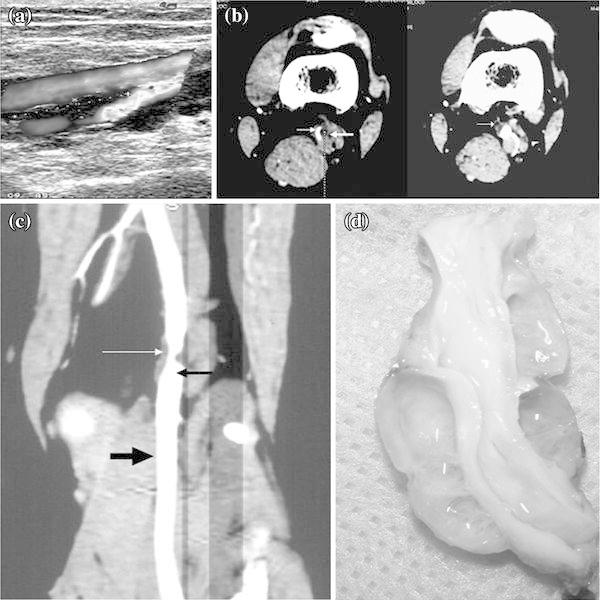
Case 1: a 49-year-old man with cystic adventitial disease (CAD) of the popliteal artery underwent surgical excision of the cyst and interposition saphenous vein bypass grafting. a Color Doppler ultrasound showed a hypoechoic cystic mass that was anterolateral to the vessel and resulted in endoluminal stenosis. b Axial computed tomography (CT) images showed a hypoattenuating mass (*thin arrowhead*) causing extrinsic compression of the popliteal artery with resultant arterial stenosis (*thick arrowhead*). c CT angiography showed a hypoattenuating mass resulting in endoluminal compression (*white arrowhead*) with a normal lumen in the distal and proximal artery (*black arrowhead*). d The excised cyst was a multilobulated pinkish-white lesion with a firm wall
